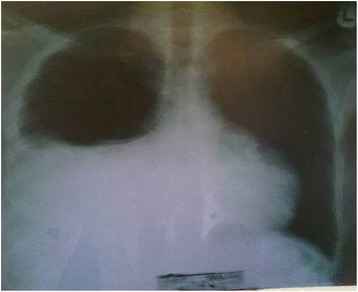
Chest X-ray showing right-sided pleural effusion and cardiomegaly
